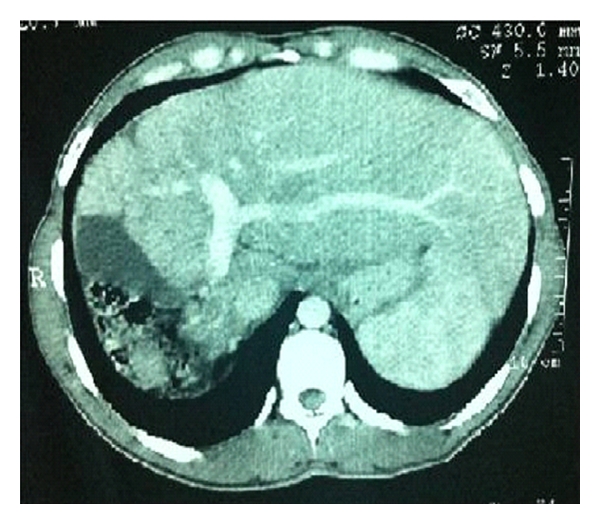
Abdominal CT: the right lobe is absent. The middle hepatic vein and extraneous segments in the left hepatic lobe are visible.
